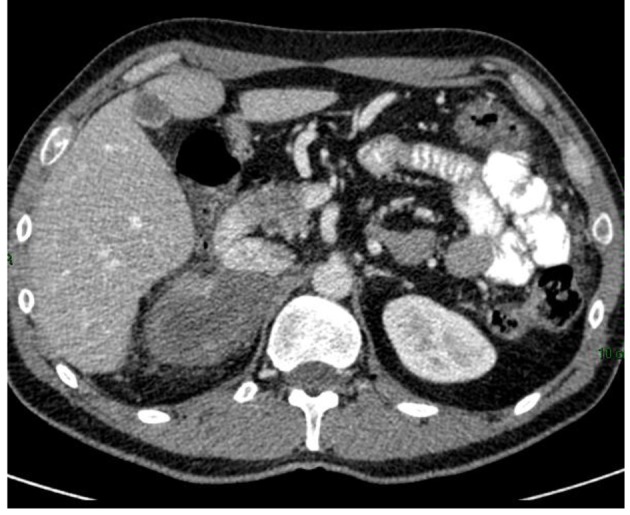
CT scan 6 months after mitotane treatment showing reduction in size of the adrenal mass.
